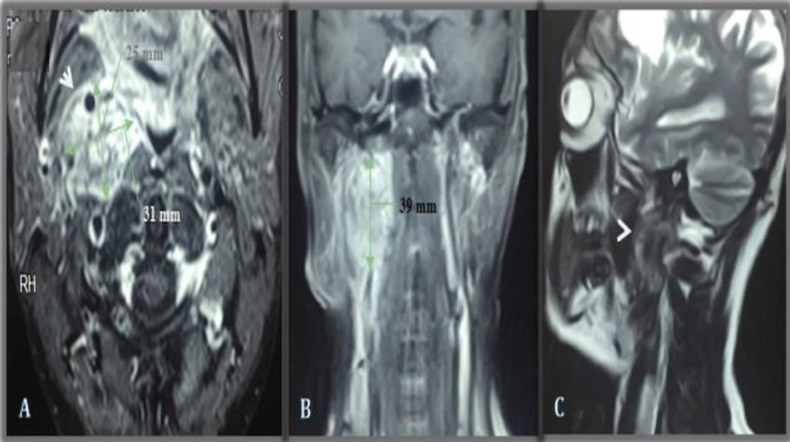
Post-treatment MRI head and neck examination with IV gadolinium contrast. (A) T1 weighted post-contrast [axial section], (B) T1 weighted post-contrast [coronal section] and (C) T2 weighted [sagittal sections]; shows almost 45% interval dec*
